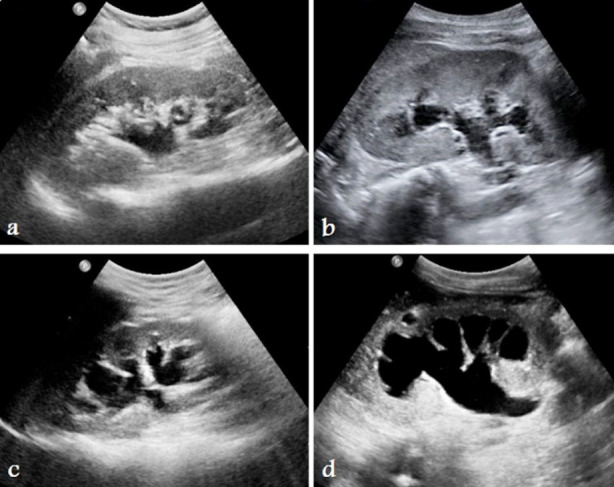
Ultrasound images of right kidney of different patients shows a) grade-1, b) grade-2, c) grade-3, and d) grade-4 hydronephrosis according to SFU grading system.
